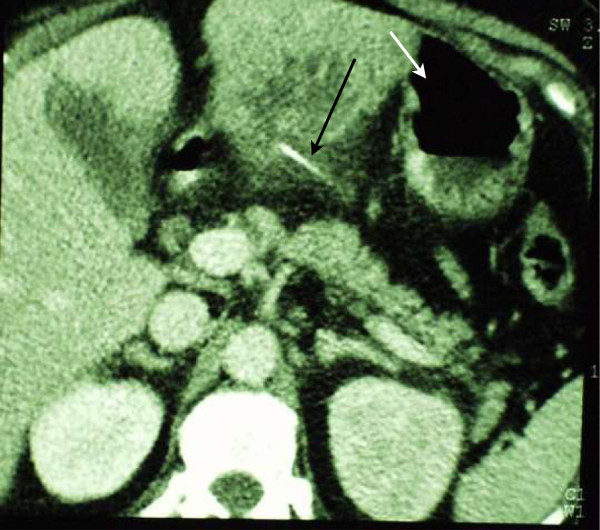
Fishbone in the centre of the abscess. Transverse abdominal CT image of the left lobe of the liver revealing the presence of a fishbone (calcified linear density, black arrow) in the centre of the abscess. Note the proximity of the stomach to the abscess (white arrow).
